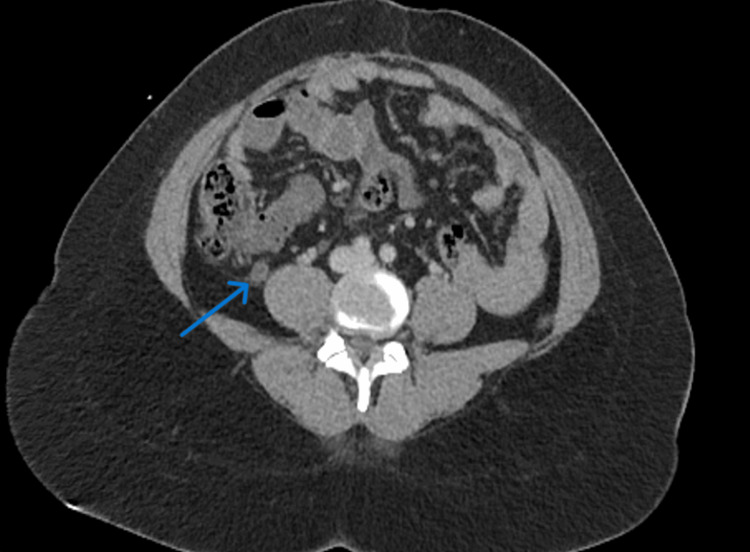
Axial computed tomography (CT) of the abdomen and pelvis demonstrating acute appendicitis. Axial CT image showing a dilated appendix (blue arrow) with surrounding periappendiceal fat stranding, consistent with acute appendicitis.
